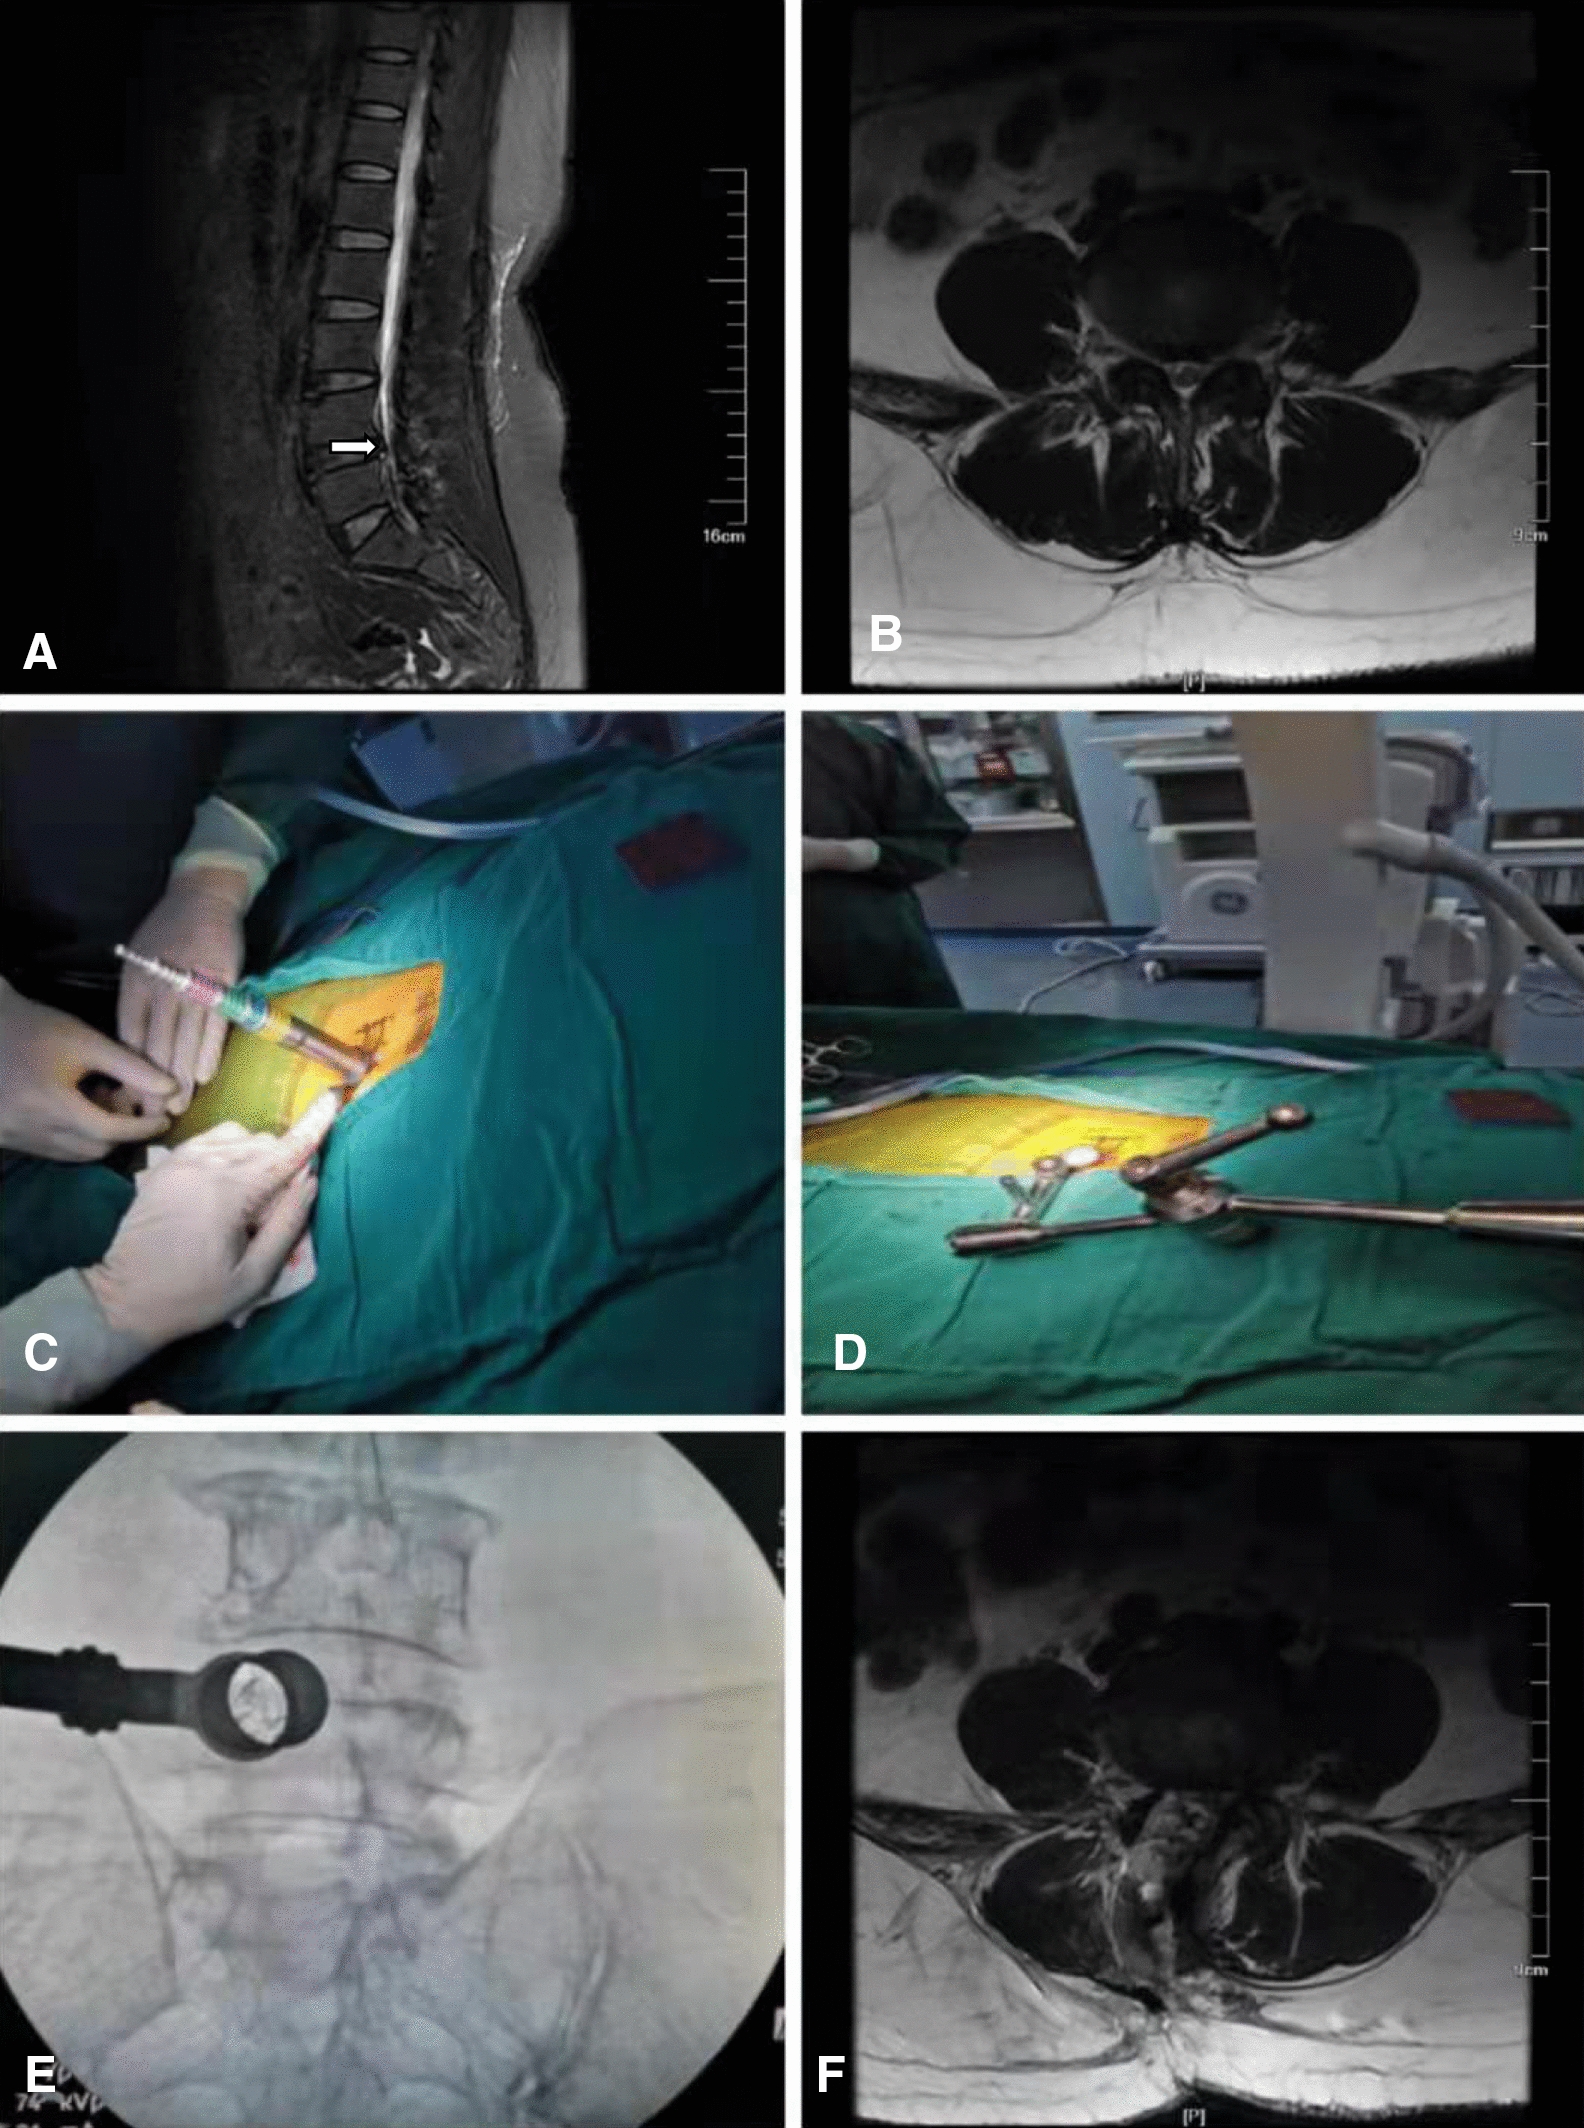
The imaging data of a 78-year-old man with LSS treated with Quadrant channel open decompression. G, H Preoperative MRI showed L4/5 spinal canal stenosis. I, J The patient's posture and operating channel and fixing devices. K Intraoperative positive and lateral X-ray showed the situation of the tube placement. L Postoperative cross-sectional CT showed adequate decompression of the L4/5 spinal canal. White arrow indicates level of decompression
